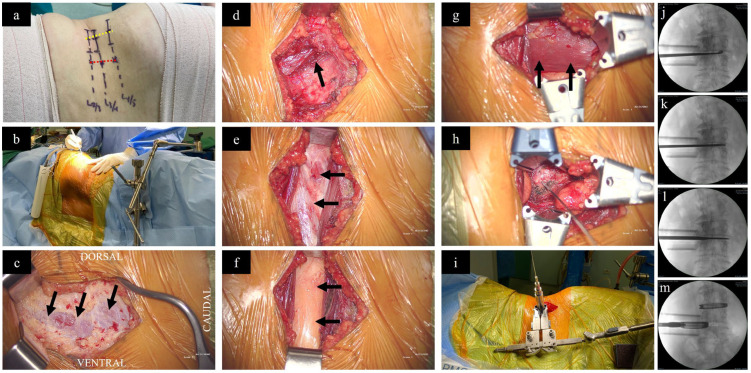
Surgical steps of OLIF. (a) While the patient is in a right lateral decubitus position, the surgical disc levels are marked (lines drawn across each disc level from anterior to posterior) under X-ray guidance. The red dotted line (3–5 centimeters anterior to the mid-disc) represents the incision site for OLIF, as performed for this patient, in relation to the yellow dotted line connecting the mid points of the disc levels, which represent the incision site for XLIF. (b) Surgeon standing on the abdominal aspect. (c) After incision of skin and subcutaneous tissue, the external oblique fascia is first encountered (arrows). (d) Following dissection of the external oblique, the internal oblique muscle is carefully split (arrow). (e) The transversalis fascia beneath the internal oblique muscle is exposed (arrows) (f) Blunt dissection of the transversalis fascia reveals the retroperitoneal fat (arrows). (g) By finger dissection, a plane is developed pushing the retroperitoneal fat anteriorly to reach the psoas muscle (arrows). (h) Placement of a guide wire into the disc space. (i) Application of the dilators and specialized retractor assembly. Preparation of the disc space using (j) curette, (k) disc punch, and (l) contralateral annular release using Cobb. (m) Placement of cages filled with bone graft under X-ray guidance.
